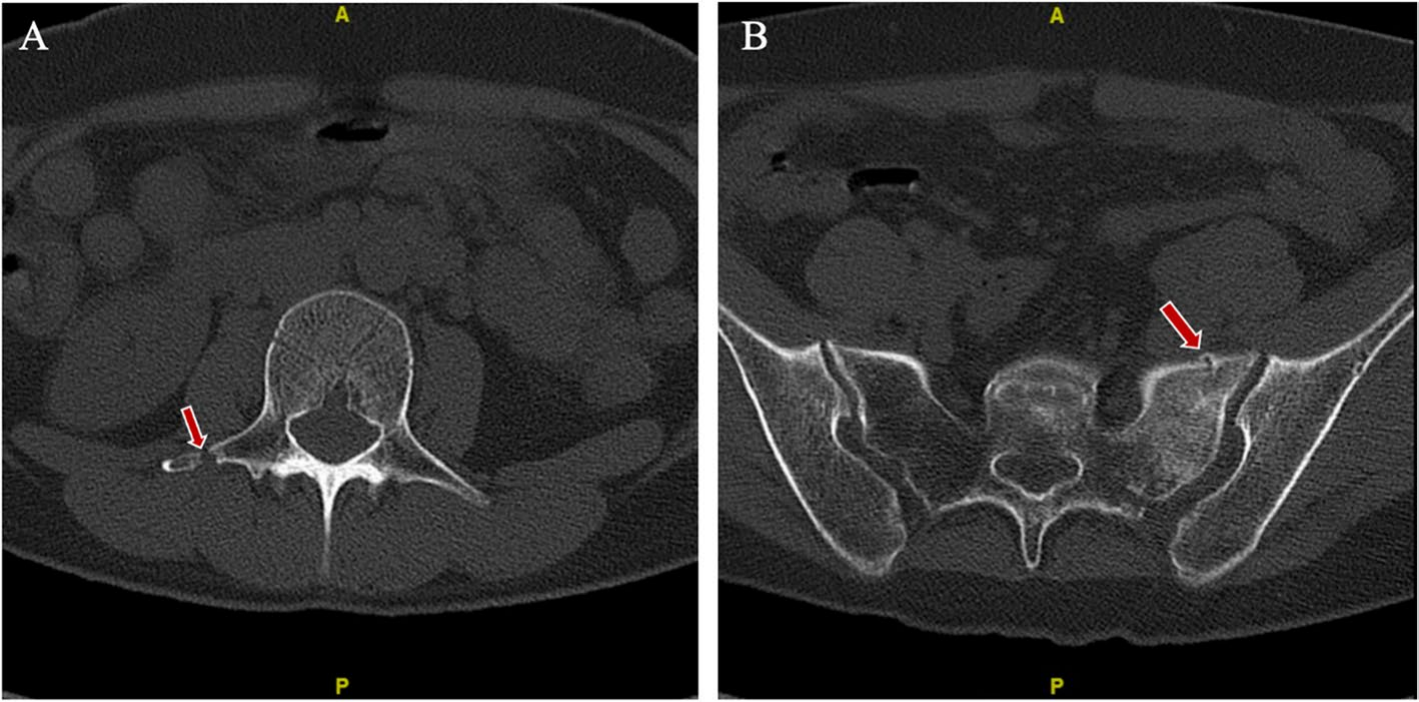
CT scan reveals fractures in L3 and the sacrum. A Fracture in the right transverse process of L3. The red arrow indicates the site of fracture. B Fracture in the left side of the sacrum. The red arrow indicates the site of cortical discontinuity and abnormal increase in bone density
